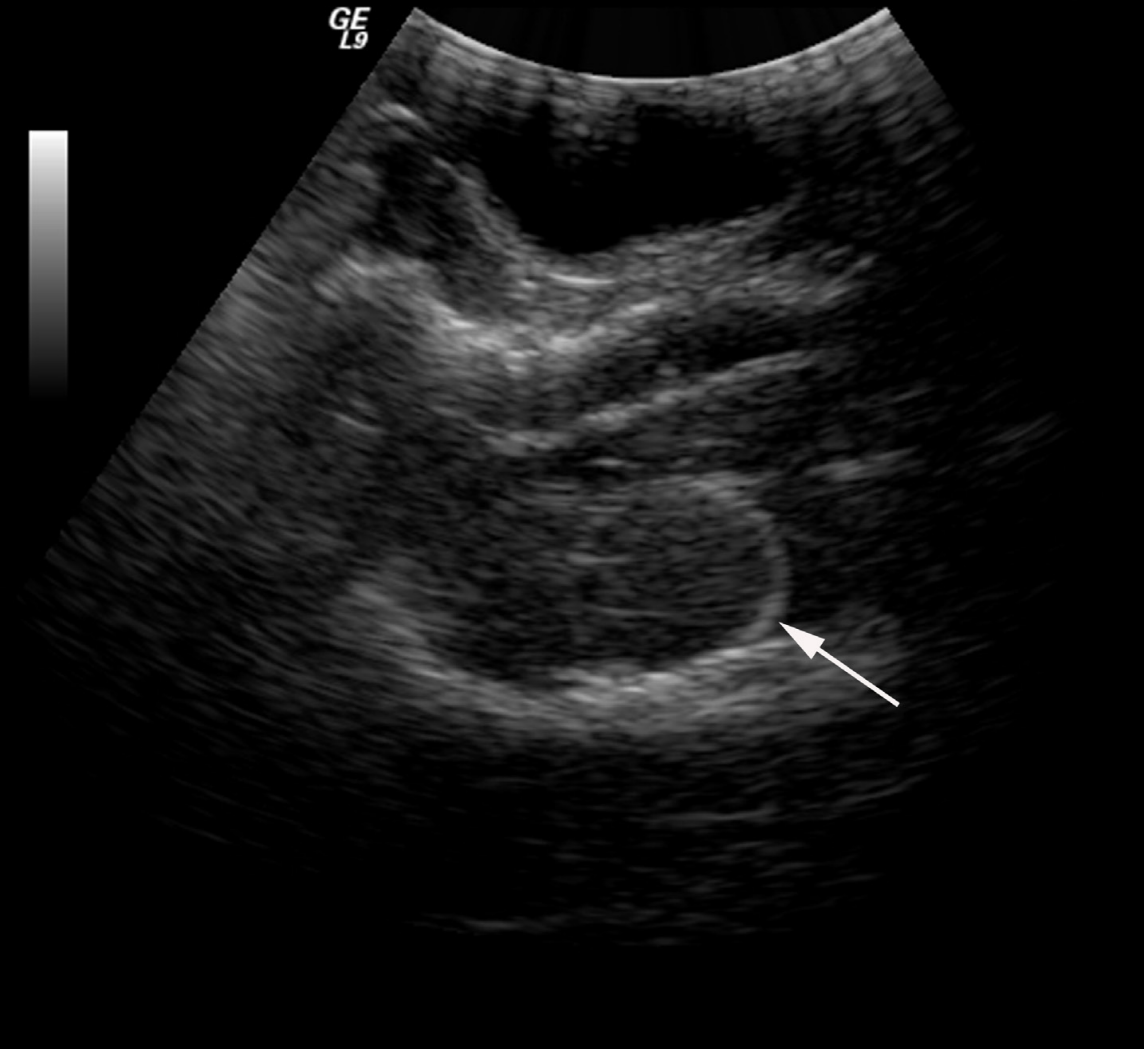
An epidural hematoma model was established, and the position of sacculus was confirmed *via* ultrasound (white arrow)
